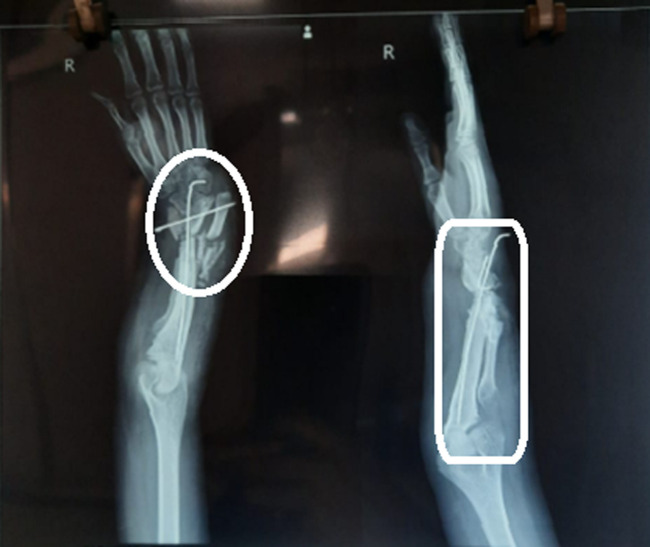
X-ray of the right side (upper limb)
